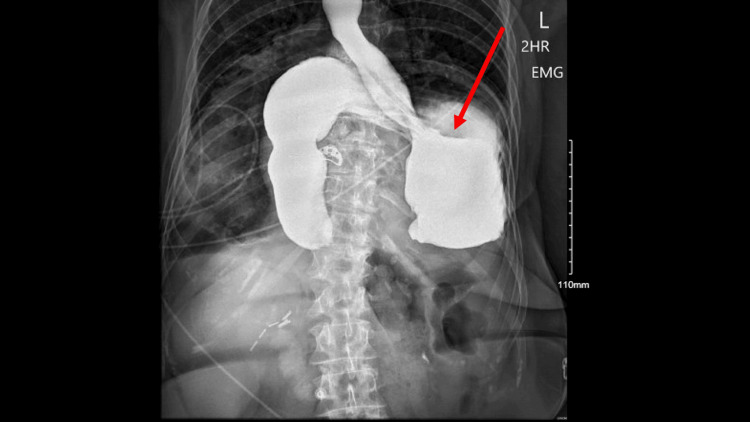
X-ray taken 7 days after readmission Findings: mesenteric axial volvulus with noted obstruction in the descending duodenum
